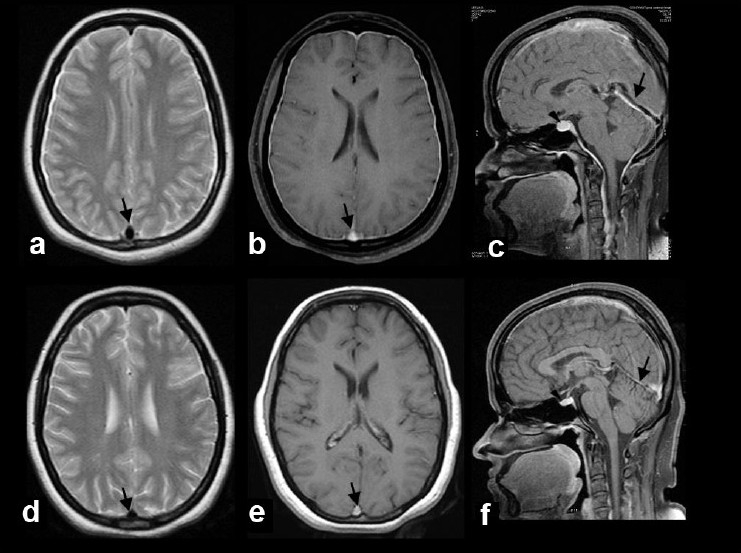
Axial T2 weighted (a), postcontrast axial (b), and postcontrast sagittal (c) images reveal distension of the sagittal sinus, straight sinus (arrows), and enlarged pituitary gland (arrow head) due to hyperemia. Axial T2 weighted (d), postcontrast axial (e), and postcontrast sagittal (f) images of a repeat MRI done after 3 months shows resolution of pachymeningeal T2 hyperintensity, gadolinium enhancement, pituitary enlargement, and venous distension
